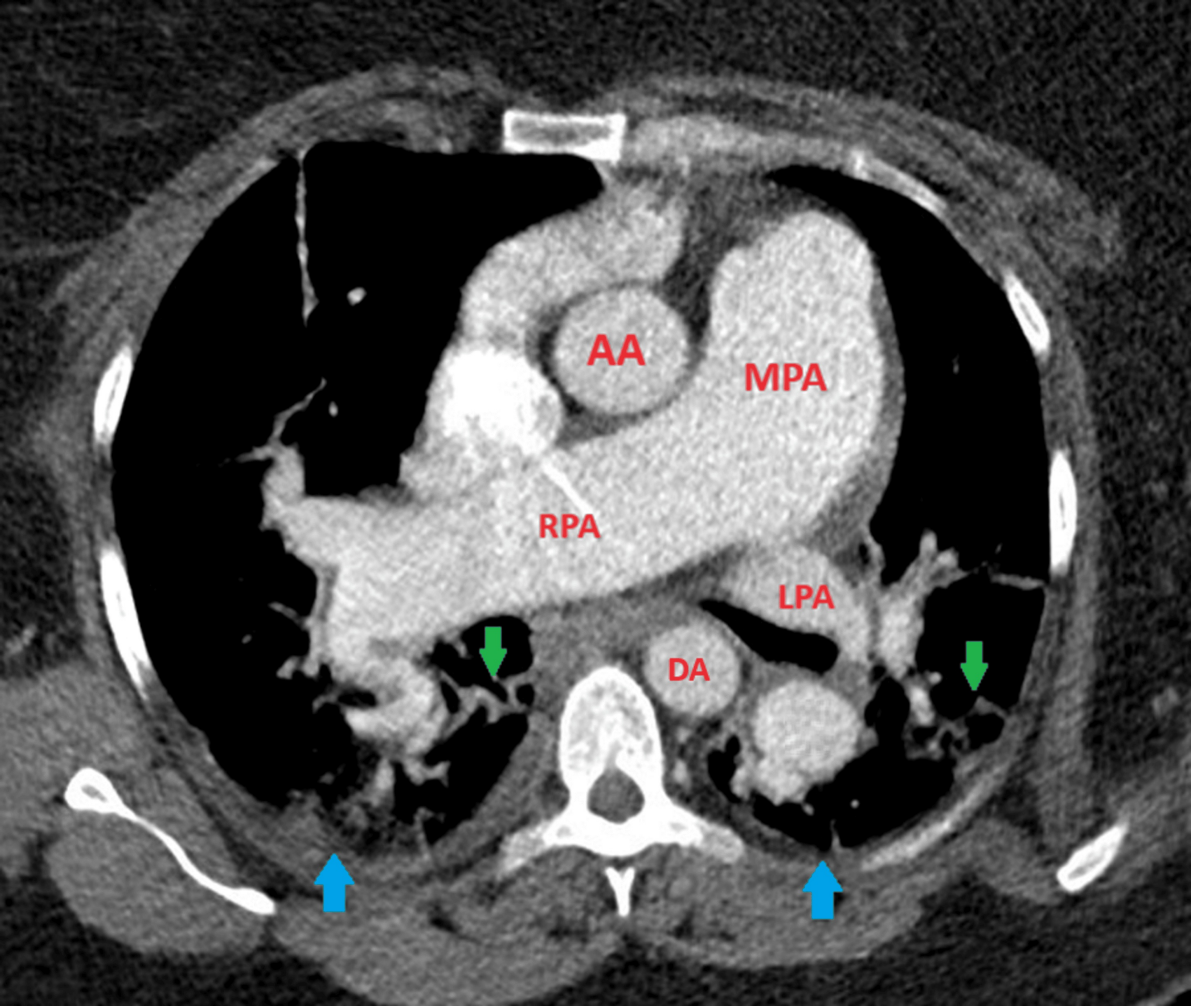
Computed tomography pulmonary angiography Computed tomography pulmonary angiography in the transverse plane reveals a patent pulmonary trunk, left pulmonary artery, and right pulmonary artery, without evidence of filling defects. There are notable bilateral infiltrates (green arrows) and small bilateral effusions (blue arrows). AA: Ascending aorta; DA: Descending aorta; LPA: Left pulmonary artery; MPA: Main pulmonary artery; RPA: Right pulmonary artery.
